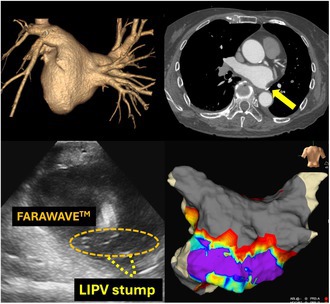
Residual pulmonary vein (PV) stumps following pneumonectomy remain electrically active and can be triggers for atrial fibrillation(AF). However, pulmonary vein isolation (PVI) in patients with severed PVs remains challenging for AF ablation. Effective radiofrequency PVI in a series of 15 cases^1^ and cryoballoon ablation in one case have recently been reported,^2^ though none with pulsed field ablation (PFA). This report provides clinical insights into the PFA using the FARAPULSE™ PFA System (Boston Scientific) for PV stump isolation. Here, we present two cases of paroxysmal AF following lower pulmonary lobectomy, in which PVI and left atrial posterior wall isolation (PWI) were performed using the pentaspline catheter under intracardiac echocardiography (ICE) guidance.
